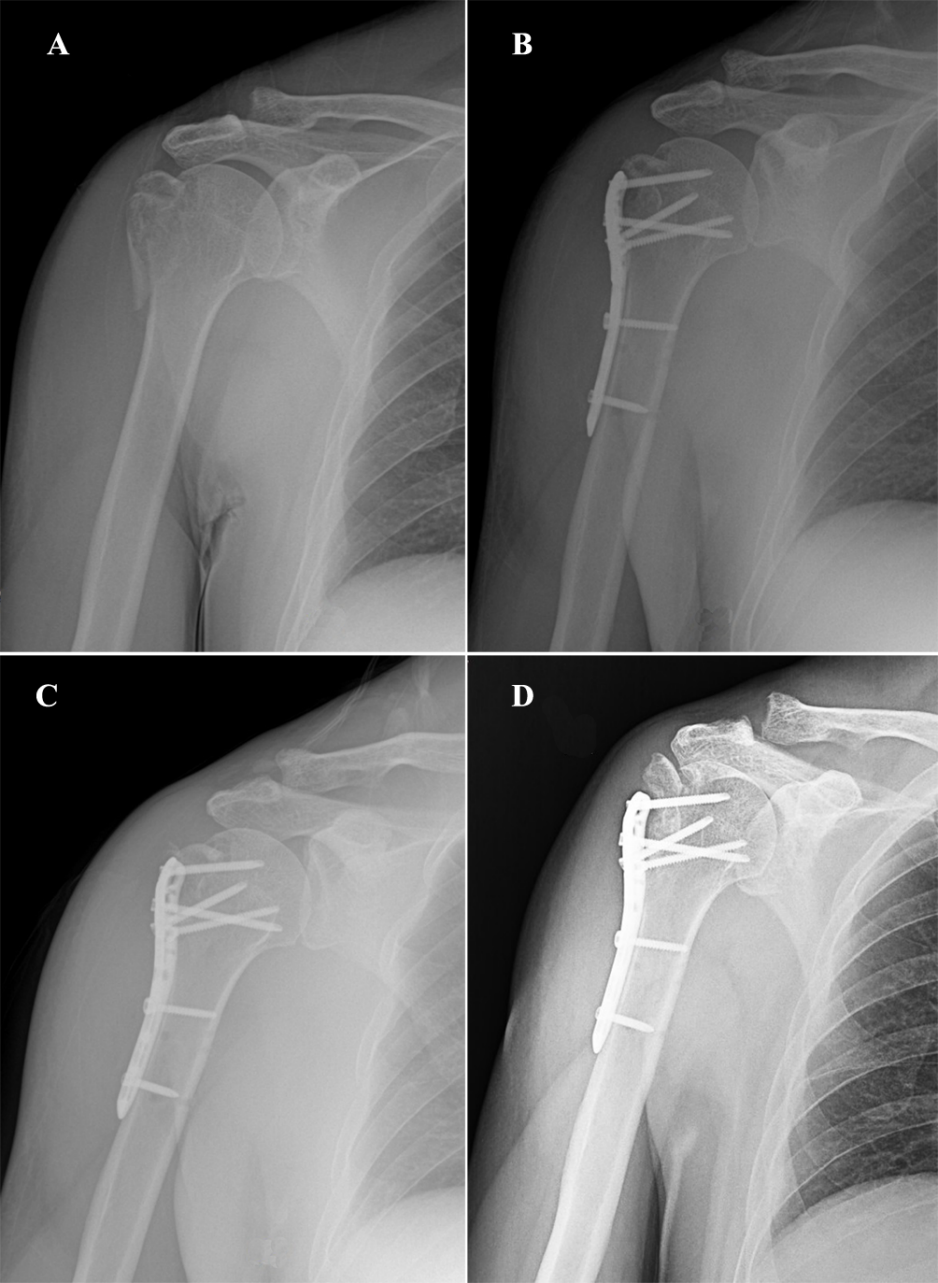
A 56-year-old male patient presenting with a comminuted fracture of the greater tuberosity of the humerus. (A) X-ray image of the shoulder joint obtained within 24 h post-injury; (B) x-ray image of the shoulder joint captured 1 day post-surgery; (C) x-ray image of the shoulder joint taken 1 month post-surgery; (D) x-ray image of the shoulder joint obtained 3 months post-surgery.
